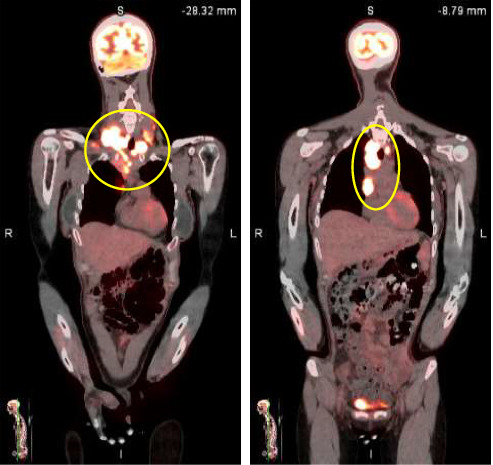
PET CT shows hypermetabolic cervical and mediastinal lymphadenopathy (yellow circles).
